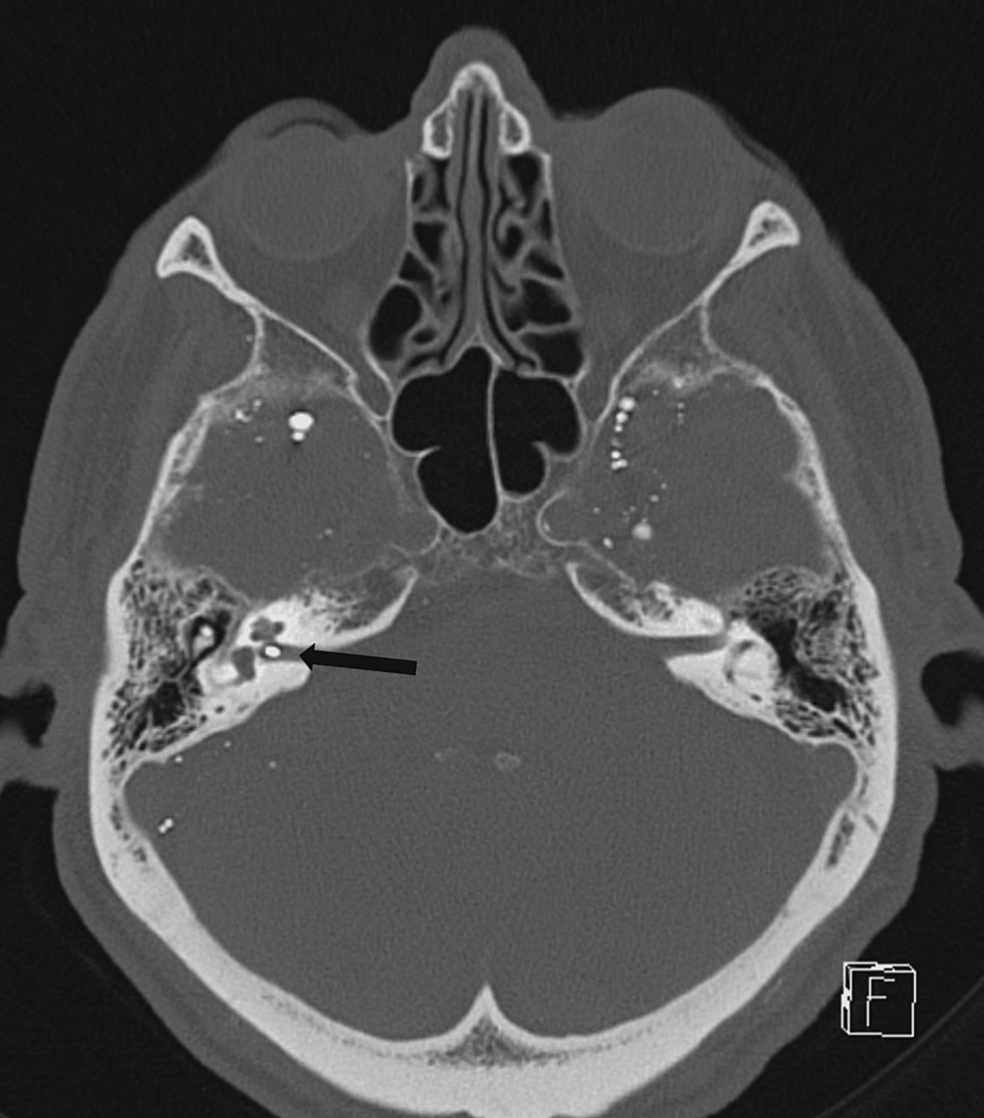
Axial non-contrast CT image of the skull. Axial non-contrast CT image of the skull showing a hyperdense droplet within the right internal auditory canal (arrow), showing that hyperdense to the adjacent petrous bone.
